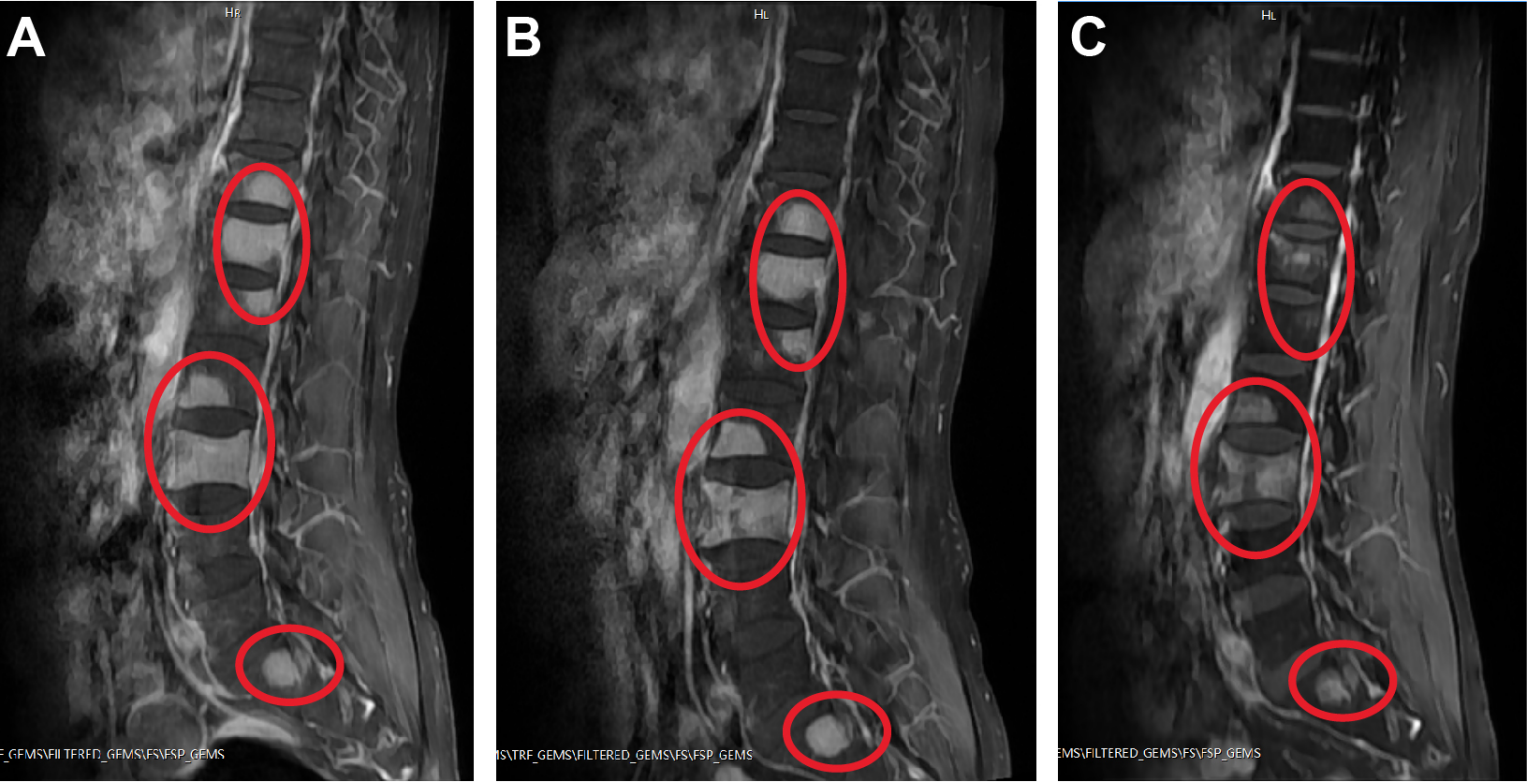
Examples of spinal sagittal T1-weighted MRI of the patient. (A) On admission. (B) After first cycle of therapy. (C) After second cycle of therapy. Abnormal high signals on T1 images are shown circled in red.
